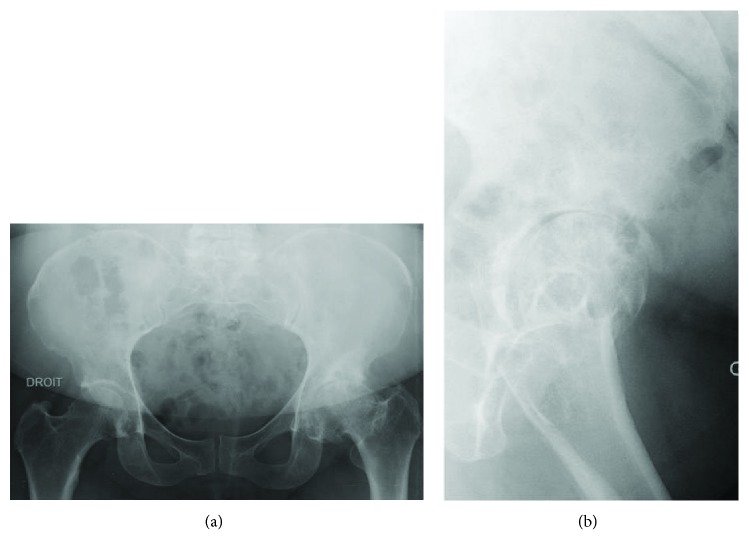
(a) Pelvic AP radiograph with left hip osteoarthritis. (b) Lateral left hip radiograph with osteoarthritis.
